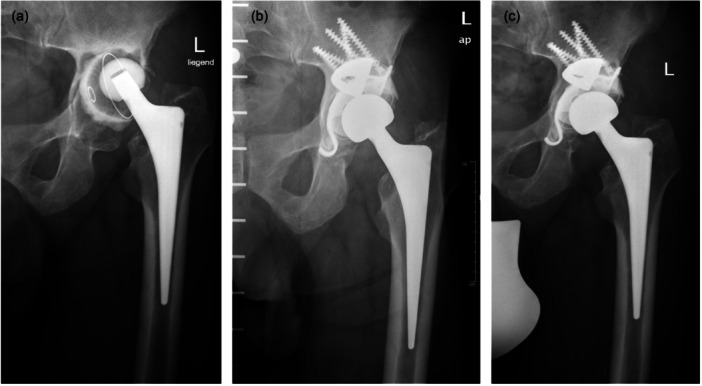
(a) Preoperative anteroposterior radiograph of the hip of a 67‐year‐old patient with severe acetabular bone loss (Paprosky type IIIa) due to aseptic loosening of the cemented cup (a). Anteroposterior radiograph of the same patient postoperative (b) and 10.8 years after surgery (c) using a modular tantalum TM buttress augment in combination with a Ganz reinforcement ring and a cemented PE cup. There are no signs of loosening of one of the components. TM, trabecular metal
